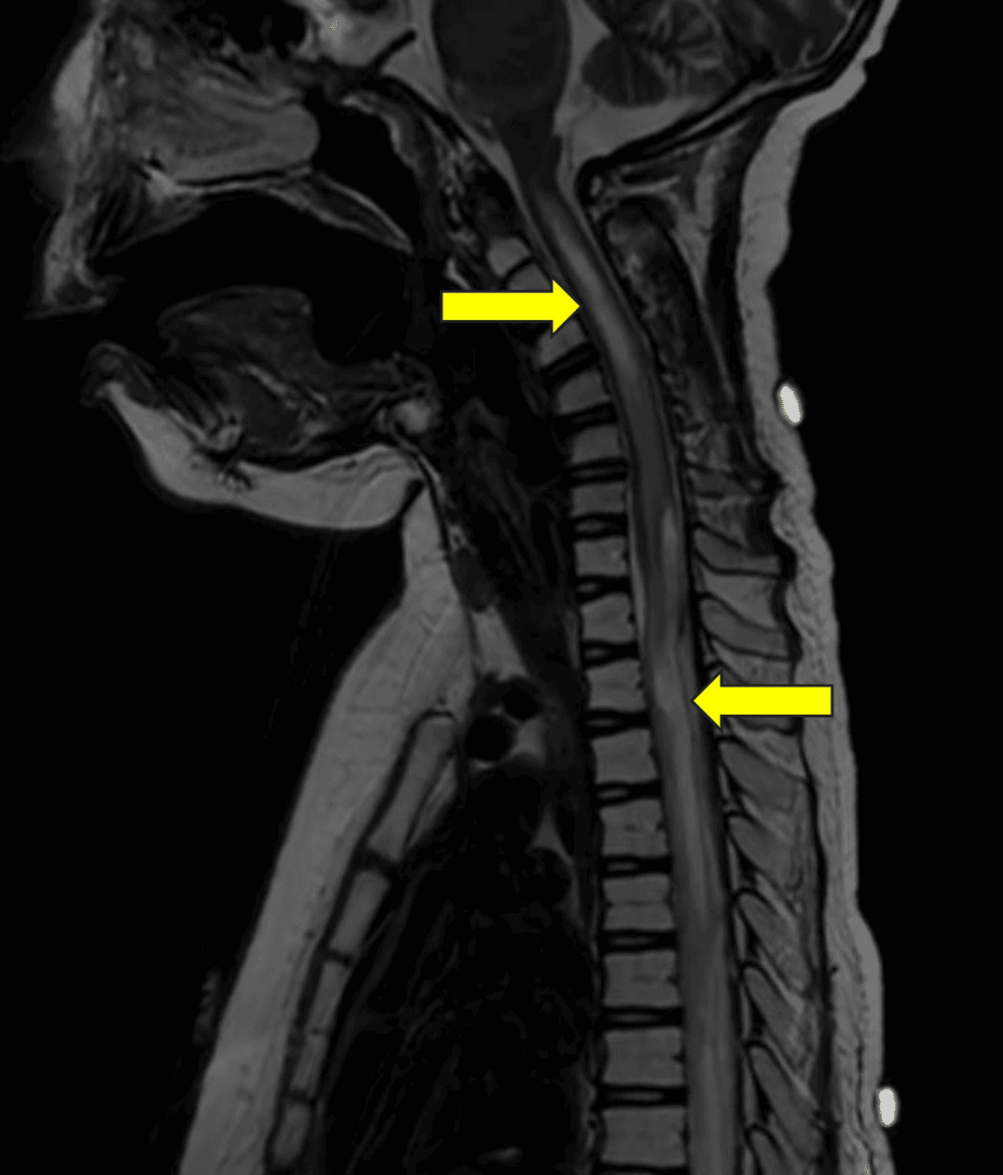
Sagittal T2 MRI showing tumor progression (yellow arrows)
